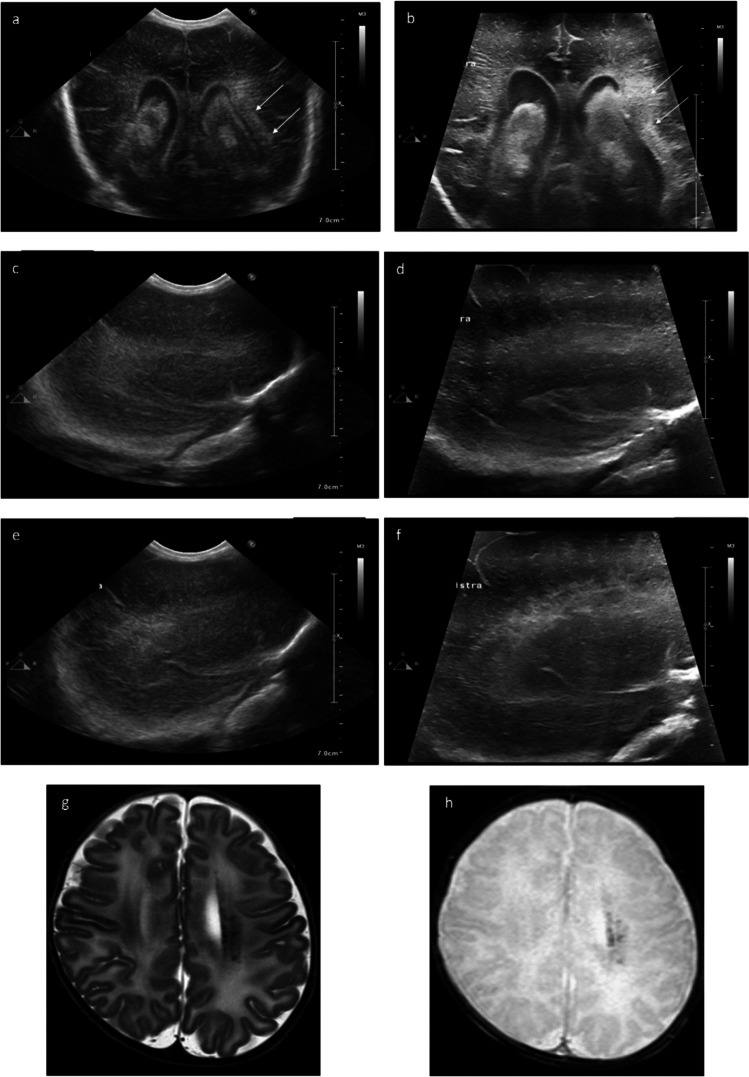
Bilateral intraventricular haemorrhage (IVH) grade 3 with post-haemorrhagic ventricular dilation and left periventricular haemorrhagic infarction (PVHI) in a preterm infant (30-week gestation, 9 days of life). a, b Coronal scans. Hyperechoic lesions involving the left periventricular white matter appear inhomogeneous (arrows) using the very high-frequency linear transducers (b) at a time when cranial ultrasound performed with the micro-convex transducer shows diffuse hyperechogenicities (a). c, d Parasagittal right scans. With the very high-frequency transducer (d), the increased echogenicity of the right periventricular white matter is better characterized as homogeneous compared to the micro-convex transducer (c). e, f Parasagittal left scans. The increased echogenicity of the left periventricular white matter (e) is similar to the right periventricular white matter (c) using the micro-convex probe. By contrast, using the very high-frequency linear transducer, increased echogenicity in the left periventricular white matter appears markedly inhomogeneous, findings compatible with PVHI (f). These hyperechoic lesions in the left periventricular white matter were confirmed as PVHI on MRI at term equivalent age (g, h). g Minute lesions with decreased intensity on T2-weighted image (axial plane) at the level of the centrum semiovale; h on T2 gradient echo image (axial plane) at the level of the centrum semiovale, localized areas of decreased signal intensity are visible in the left periventricular white matter
